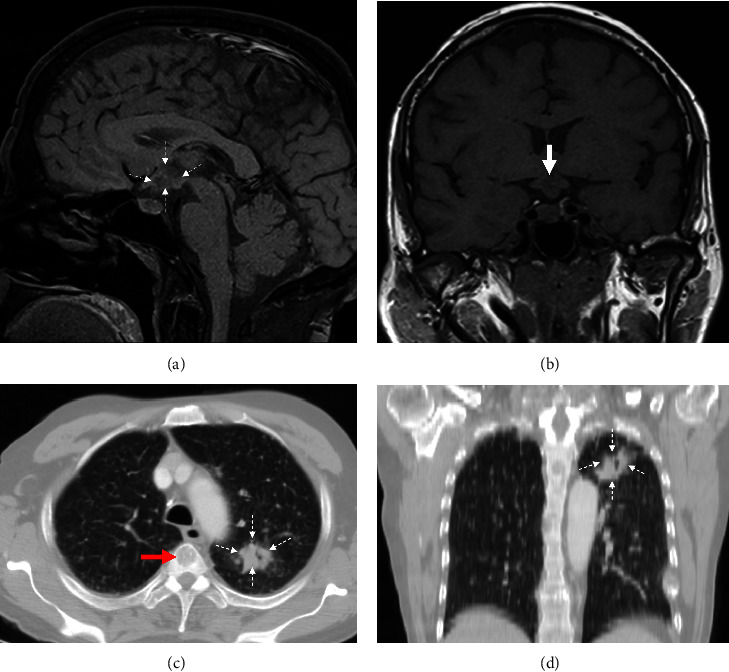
(a) The patient's sagittal MR image without contrast for the pituitary; the dashed arrowhead points to the hypothalamic mass. (b) Coronal image without contrast shows the optic chiasm and pituitary stalk infiltration. (c) Axial lung window shows the apical lung mass (the dashed arrowheads), and the red arrow points to an osteolytic lesion within the vertebral body. (d) Coronal lung window shows the apical lung mass (the dashed arrowheads).
